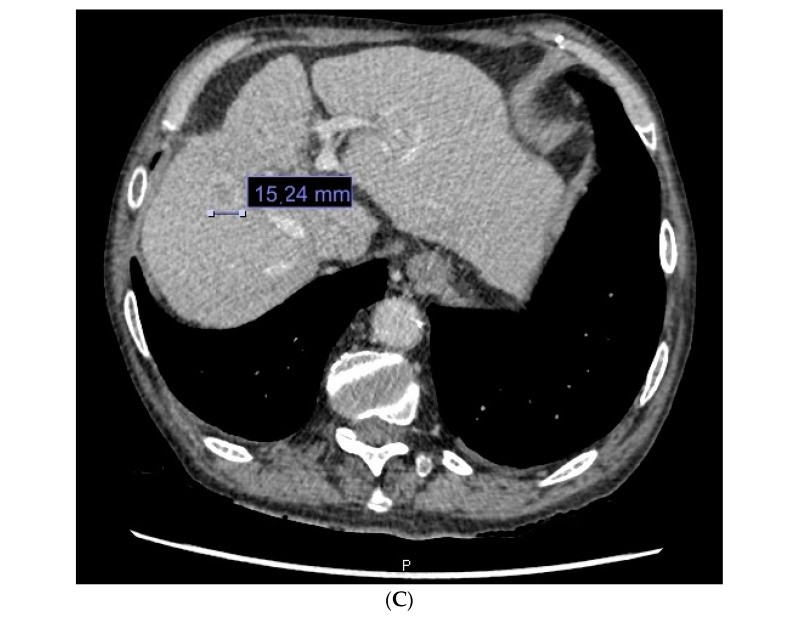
Diagnostic images of HCC. In image (A), a hypoechogenic lesion of 13 mm is observed on cirrhotic liver using ultrasound. Image (B) displays the arterial phase of the subsequent triphasic CT scan, revealing an image slightly larger than previously described. Finally, image (C) shows the venous phase with the characteristic washout, confirming the diagnosis of HCC. HCC: hepatocellular carcinoma. CT: computed tomography.
